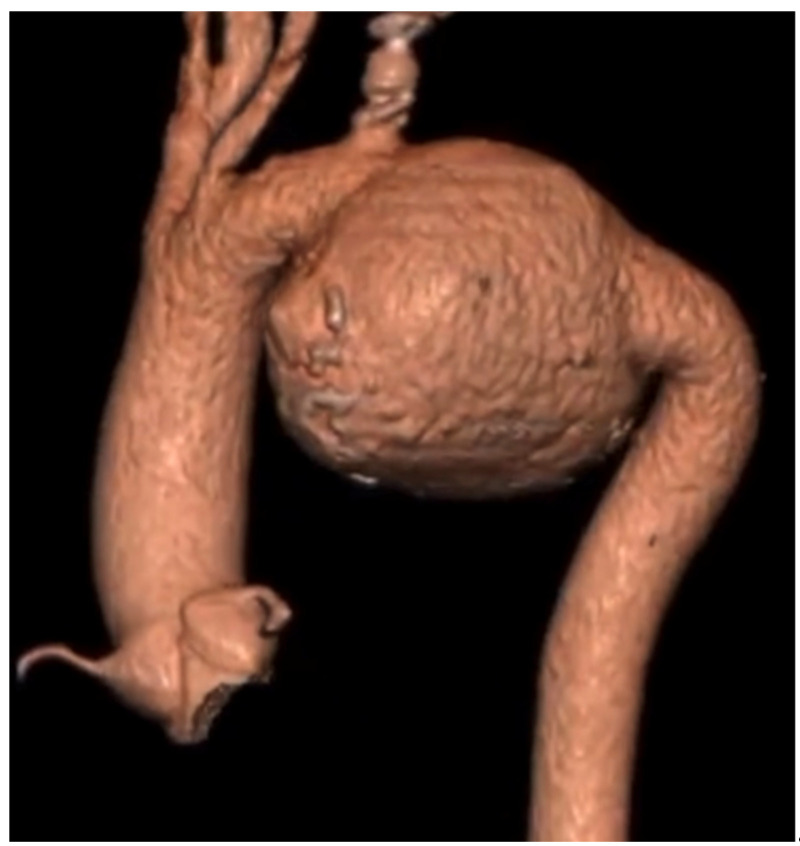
Three-dimensional volume-rendered reconstruction of the pre-operative CTA showing the 8.5 cm aortic pseudoaneurysm.
